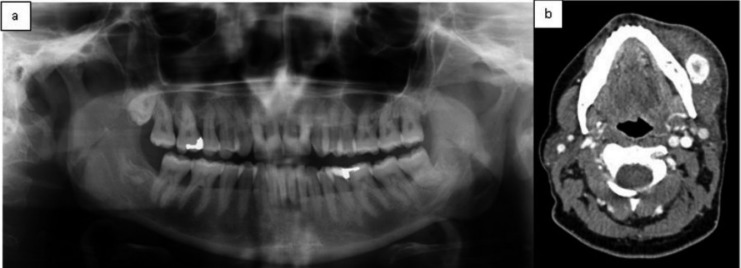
Radiological study. a) orthopantomography. b) CT scan: well-defined calcification located within masseter muscle.
